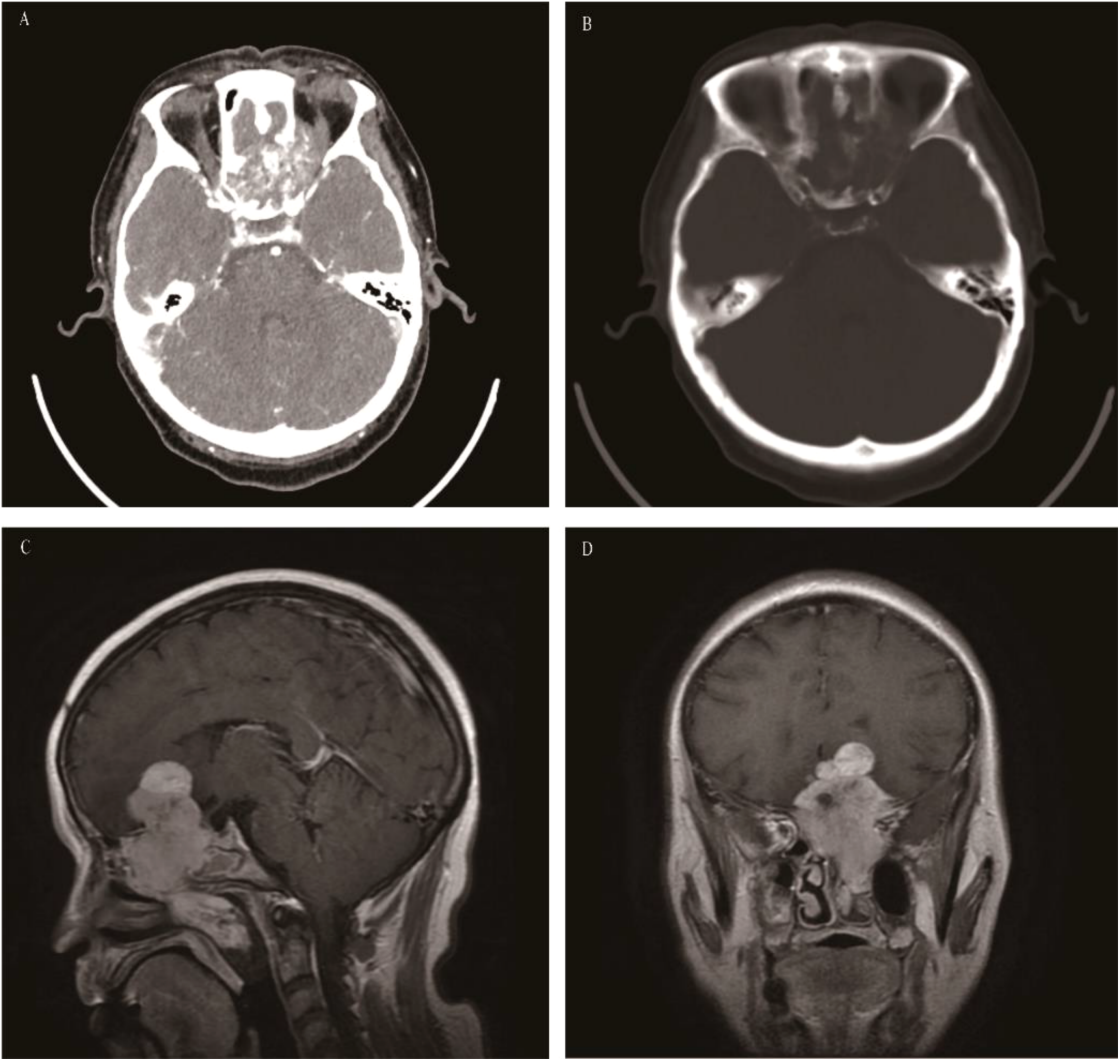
Osteosarcoma: Axial CT showed a mass in the left ethmoid sinus, which involved the left orbit and the intracranial region. Tumor bone was seen inside the mass, and there was obvious enhancement on contrast-enhanced CT (A, B). Sagittal and coronal contrast-enhanced MRI showed obvious enhancement, and the tumor bone within the tumor presented as low signal (C, D).
